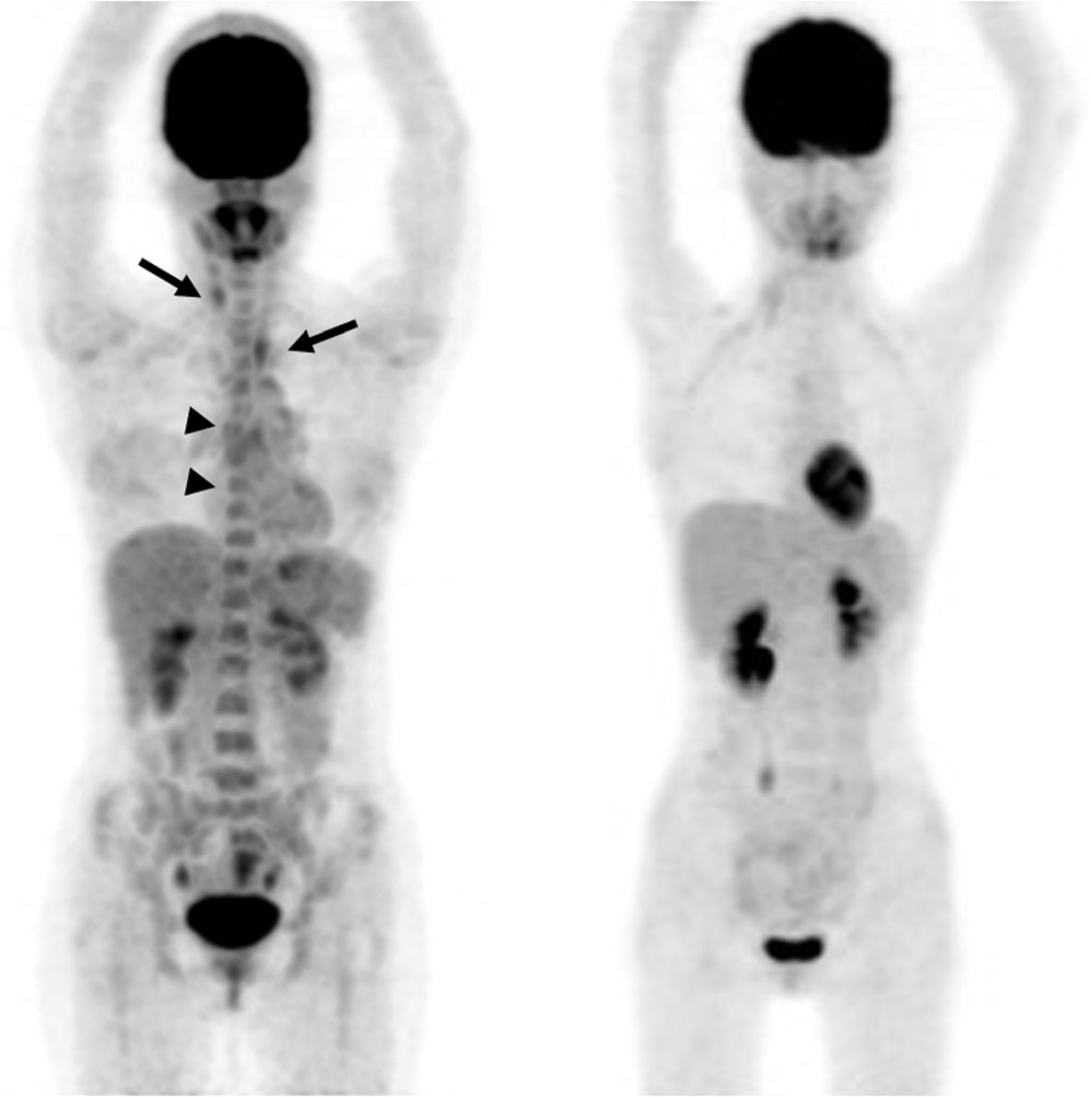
[F-18]FDG PET/CT scan of an 18-year-old woman diagnosed with Takayasu arteritis and an age-matched control patient. [F-18]FDG uptake is present in the right and left common carotid arteries and aortic arch (arrows). Note the [F-18]FDG uptake in vertebrae and pelvic bones (arrowheads), compared with the control
